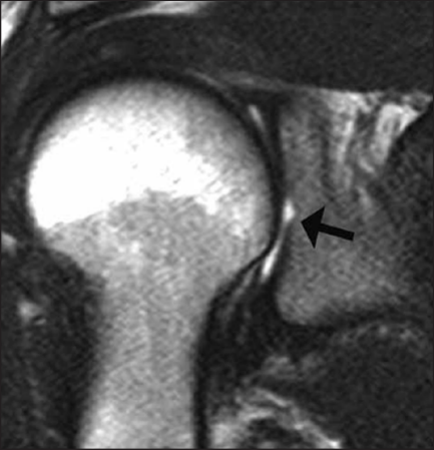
Bare area of the glenoid on MRI. Coronal oblique PD-weighted MR image displays a defect in the cartilage filling up with a moderate amount of joint fluid (arrow) without any thickening of the subchondral bone. As for the tubercle of Assaki, the bare area of the glenoid may be mistaken for a cartilage ulceration.
